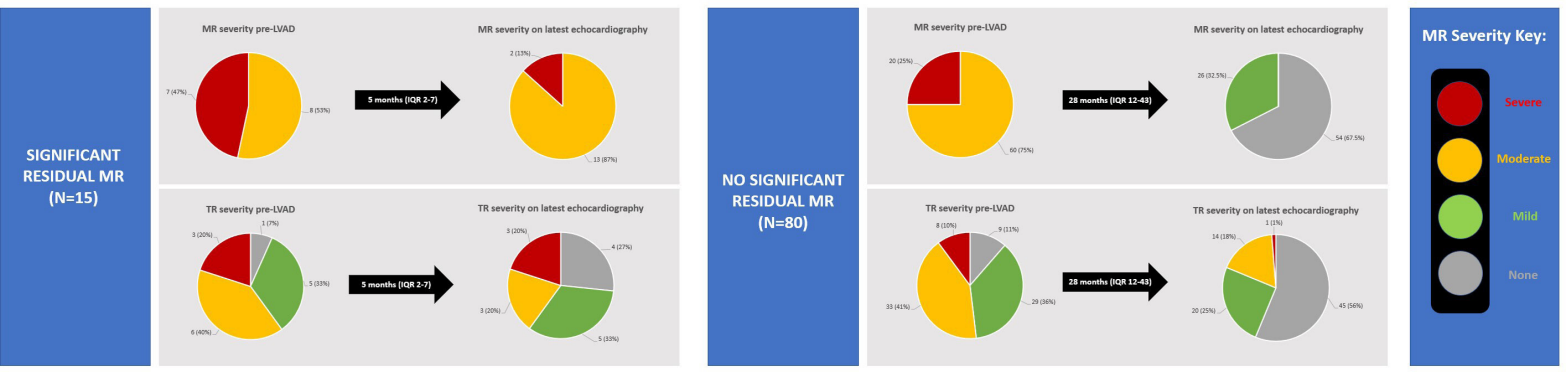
Pie charts showing the severity of MR and TR in patients with significant residual MR after LVAD implantation. Significant residual MR was defined as ≥ moderate severity. This analysis included only patients with ≥ moderate MR at baseline who had follow-up echocardiography. LVAD, left ventricular assist device; MR, mitral regurgitation; TR, tricuspid regurgitation.
